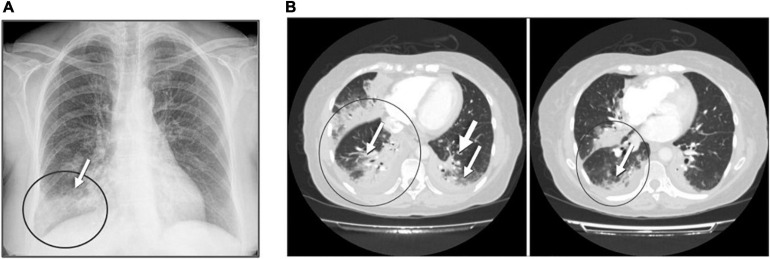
Admission chest radiography and computed tomography of the patient with szpneumonia caused by *L. feeleii*. (A) Chest radiography: radiological signs showed condensation in the lower right lobe (arrow) and a bilateral interstitial pattern. (B) Computed tomography showed extensive areas of consolidation with air bronchogram. Predominant affectation was observed in posterior basal segments and pseudo-nodular opacities of acinar aspect (arrows).
